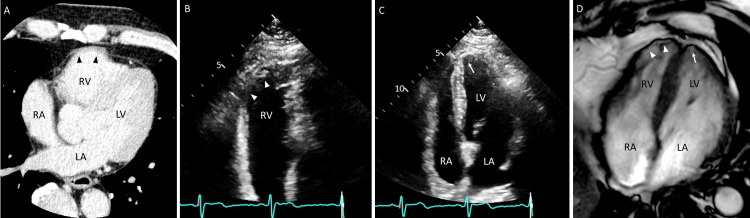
Cardiac imaging Computed tomography of the heart shows bulging of the right ventricular free (RV) wall (A, arrowheads). Repeat echocardiography shows asynergy not only in the RV free wall (B, arrowheads) but also in the left ventricular (LV) apex (C, arrow). Cardiac magnetic resonance shows partial thinning in the same regions of both RV and LV (D, arrowheads and arrow, respectively). LA indicates left atrium; RA, right atrium.
